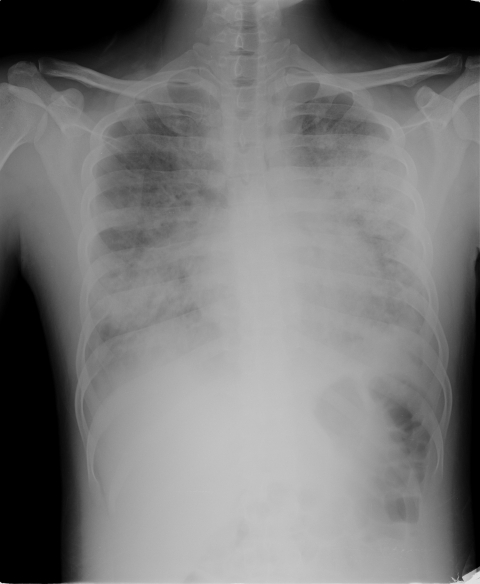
Radiograph (anteroposterior view) of patient with acute respiratory distress syndrome and oseltamivir-resistant pandemic (H1N1) 2009 virus.
